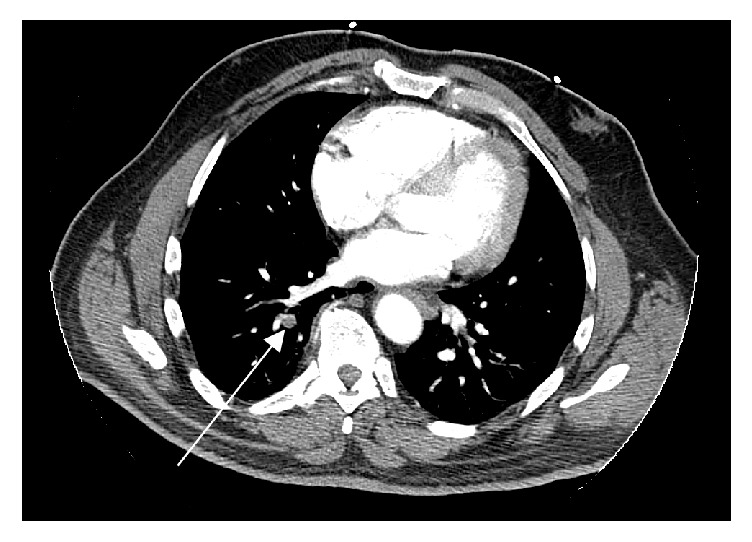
High-resolution CT scan of the thorax with intravenous contrast on day 2: the white arrow shows right lower lobe pulmonary embolism.
